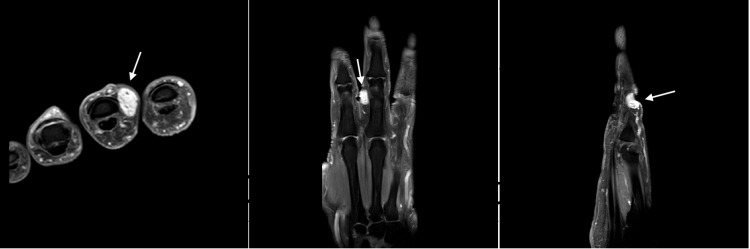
MRI T1 axial (left), coronal (middle), and sagittal (right) sections of the hand showing hyperintense lesion of the radial aspect of the middle finger. The arrows on the different cuts show the location of the mass on the middle finger.
